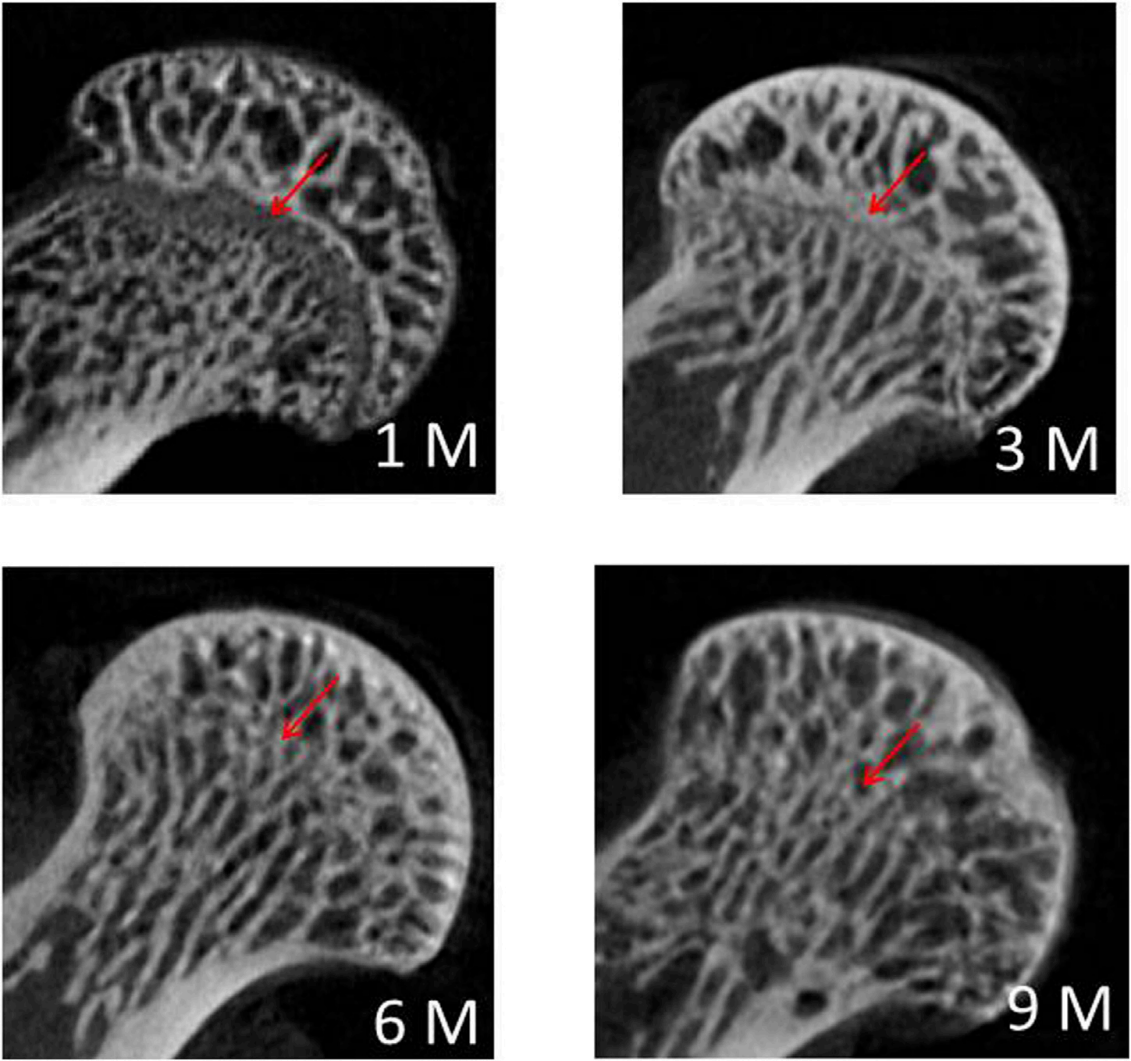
Typical radiographic images of the proximal femurs at 1 M, 3 M, 6 M and 9 M (red arrow: growth plate).
